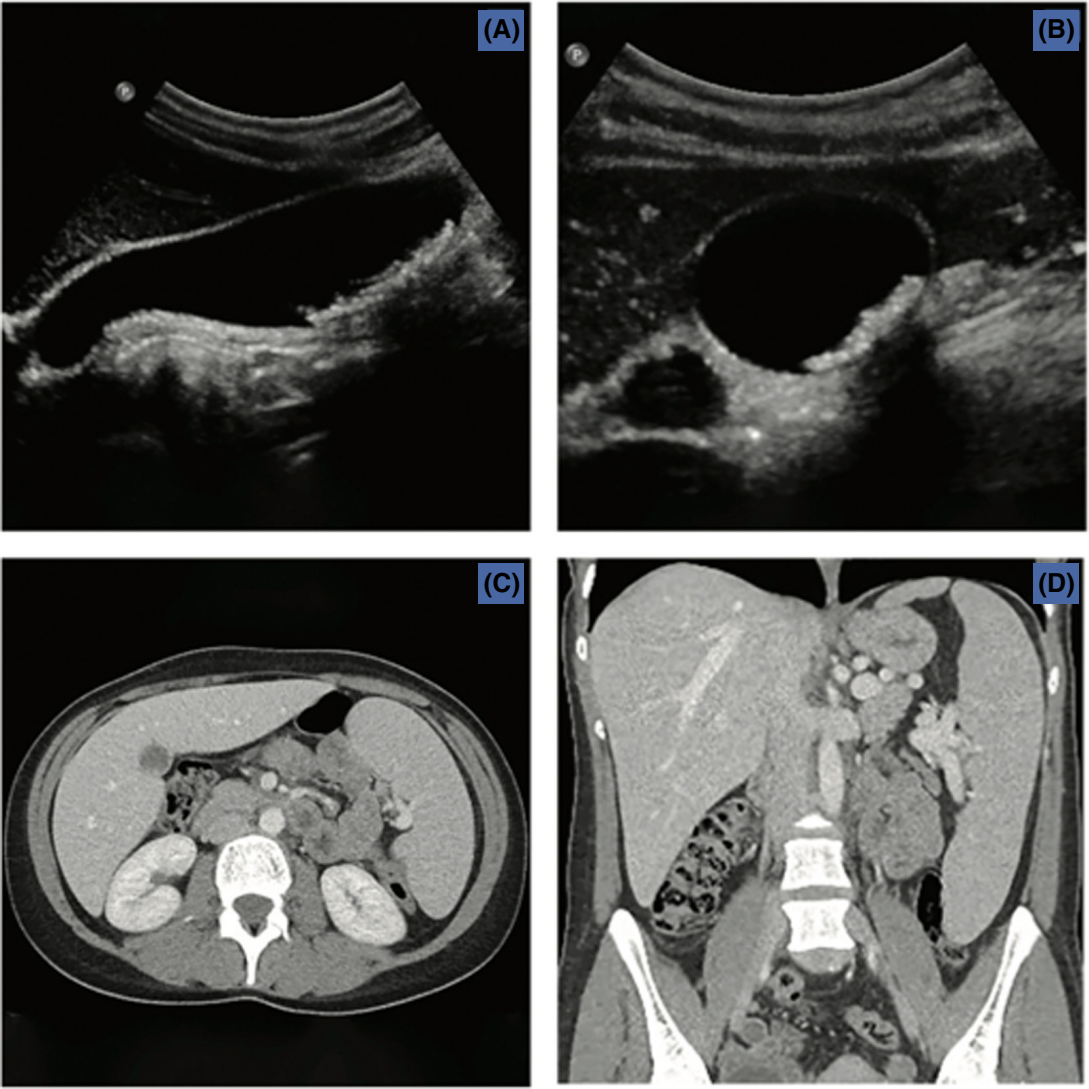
Imaging Studies (A) Ultrasound long‐axis view—cholelithiasis; (B) Ultrasound short‐axis view—cholelithiasis; (C) Abdominal computed tomography (CT) transverse—splenomegaly; (D) Abdominal CT coronal—massive splenomegaly
